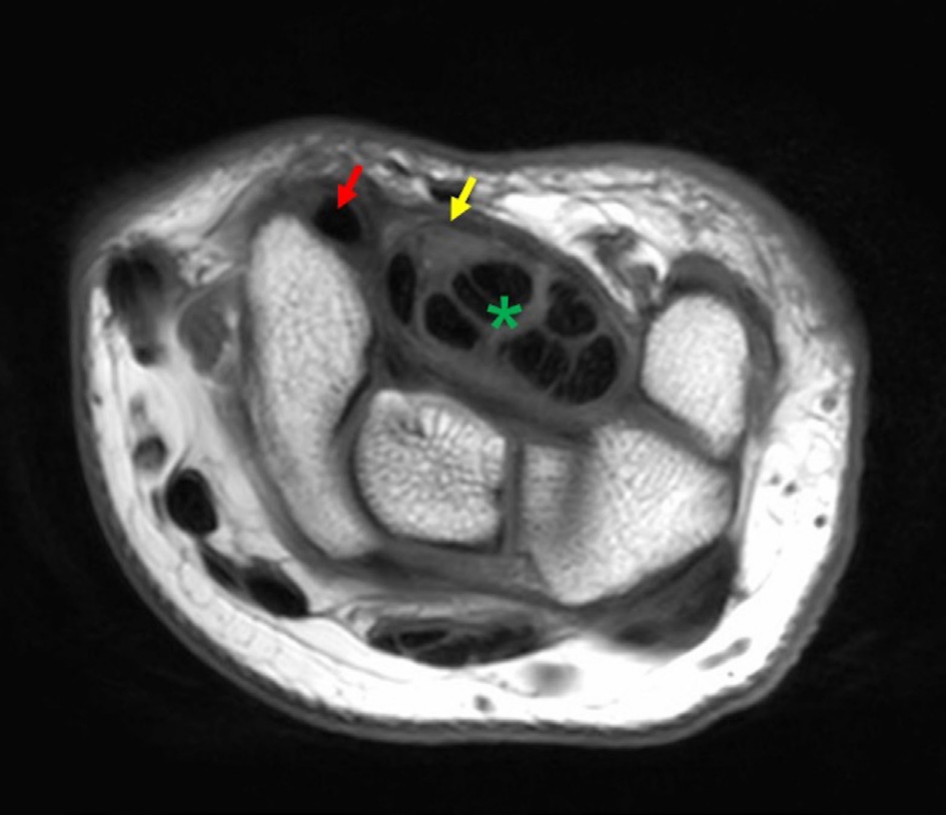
An MRI section demonstrating flexor carpi radialis tendon’s relation with the carpal tunnel. Red arrow; the tendon of flexor carpi radialis. Yellow arrow; flexor retinaculum. Green asterix; tendons of flexor dig
